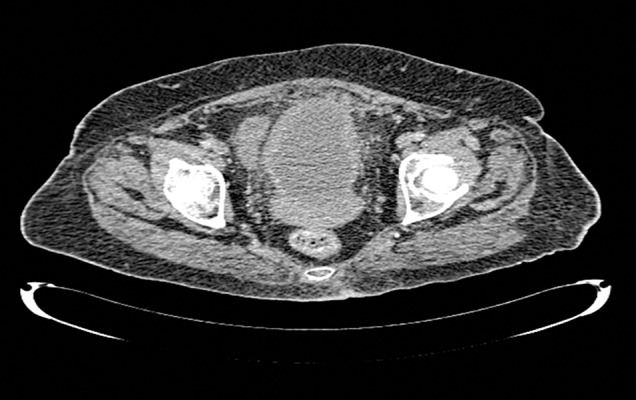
CT-Scan: Mass, a 14 × 11.5 cm cystic lesion in pelvic cavity without significant change in post-contrast images.
